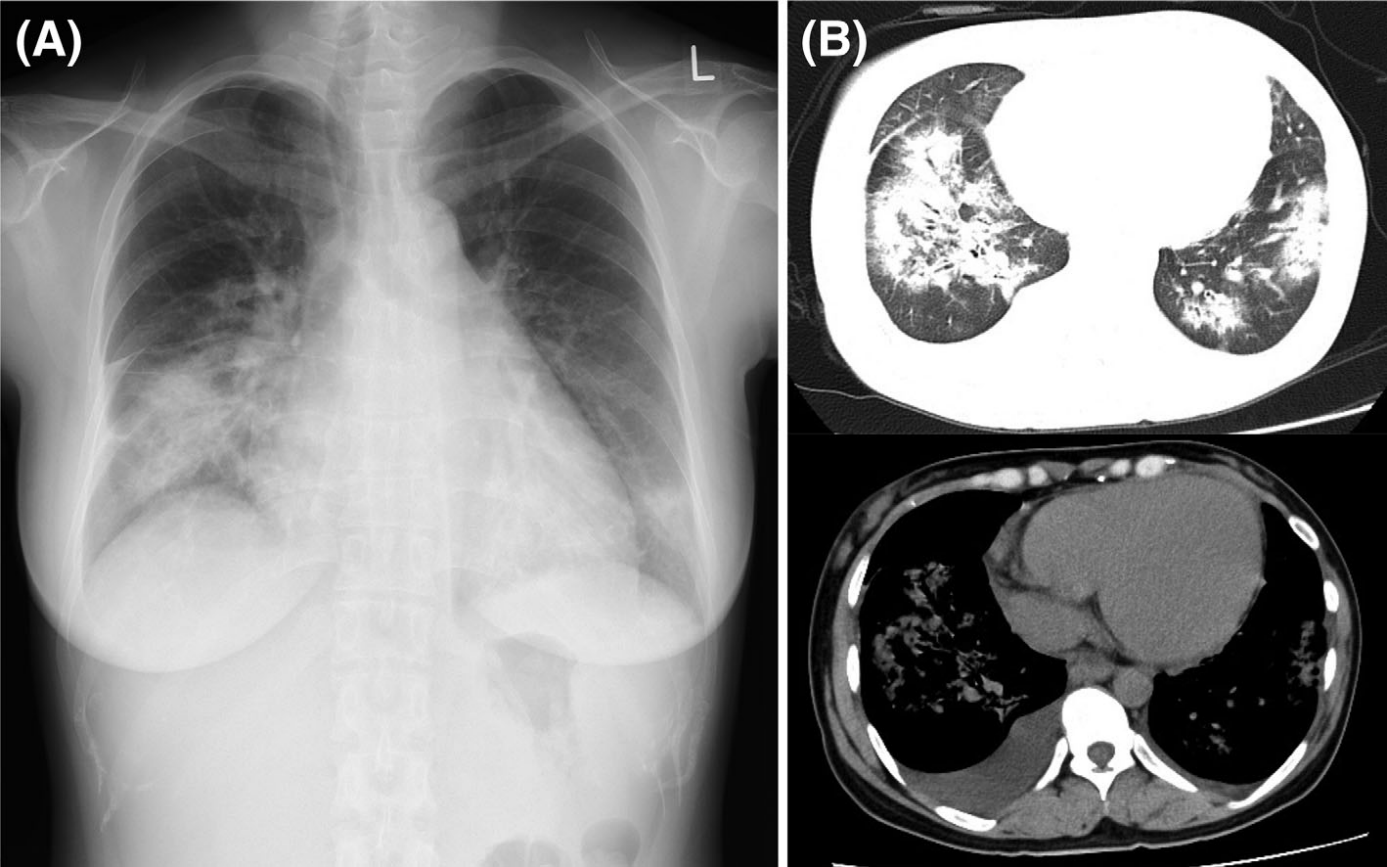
Imaging findings. (A) Chest radiograph showing remarkable cardiac enlargement and infiltrating shadows in the lung fields. (B) Chest computed tomography showing bilateral pulmonary consolidation and right pleural effusion
